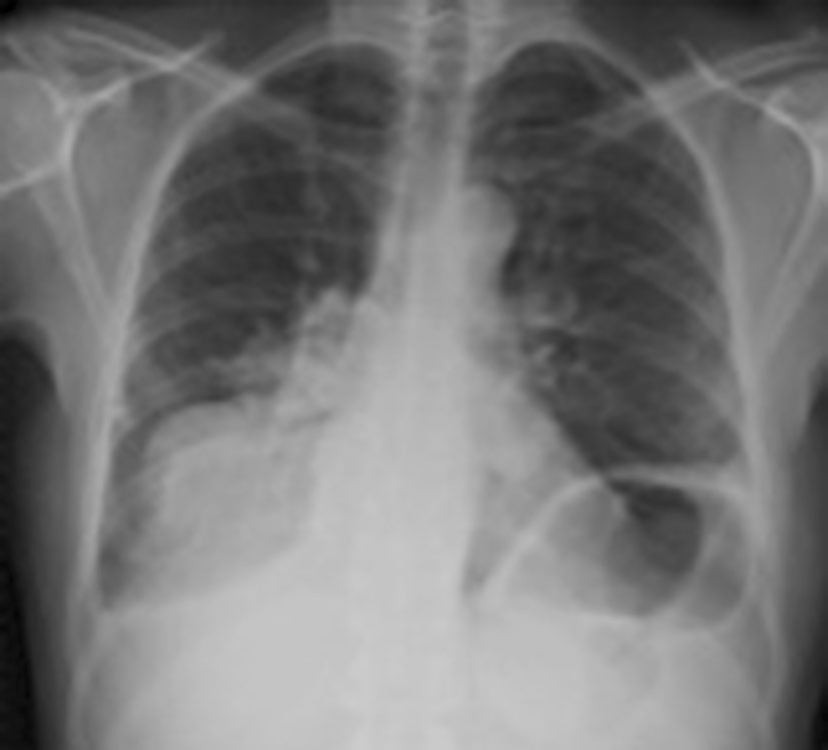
Chest radiograph with consolidation adjacent to the right hemi-diaphragm
